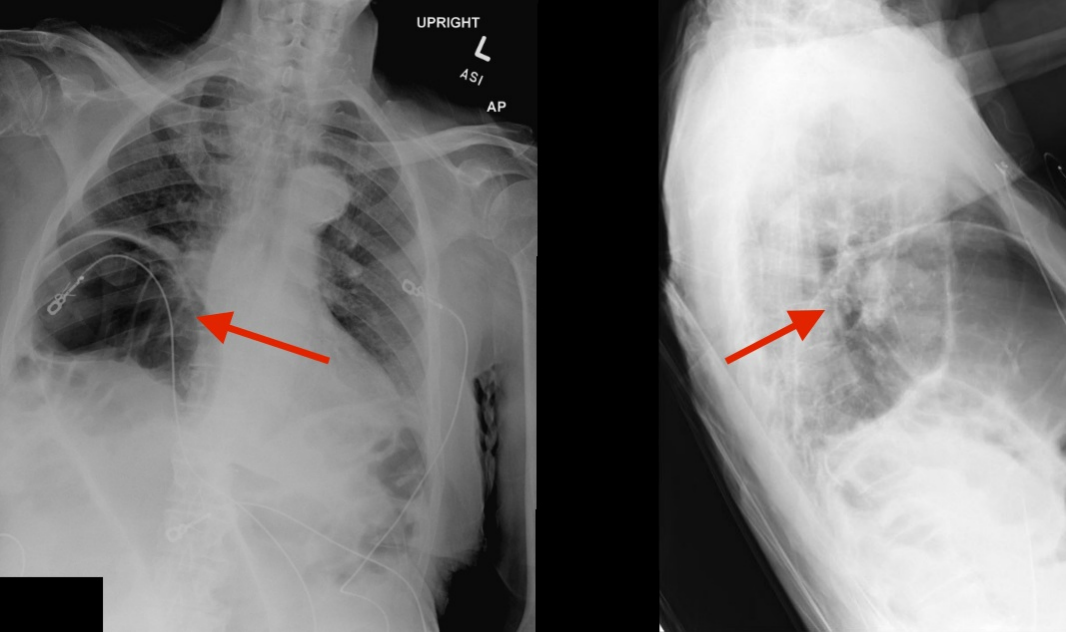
Chest radiograph (anteroposterior and lateral views) showing interposition of the hepatic flexure of the colon between the diaphragm and the liver (Chilaiditi's sign).
